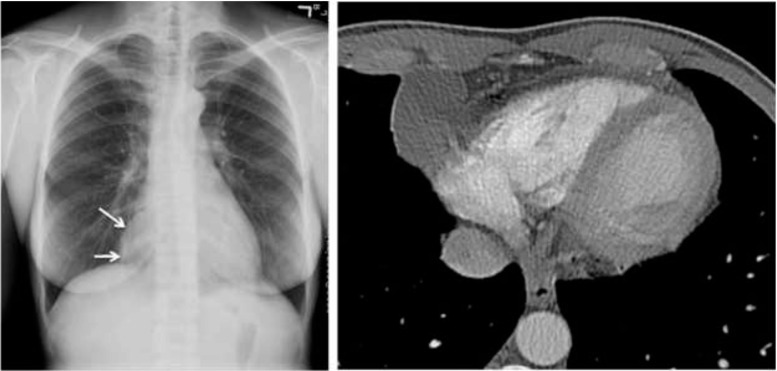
Pericardial cyst. (*left*) anterior chest X-ray suggestive of a mass at the right costophrenic angle. (*right*) contrast cardiac CT demonstrating that the mass correlates to a homogenous cyst in the right cardiophrenic angle most consistent with a benign pericardial cyst
